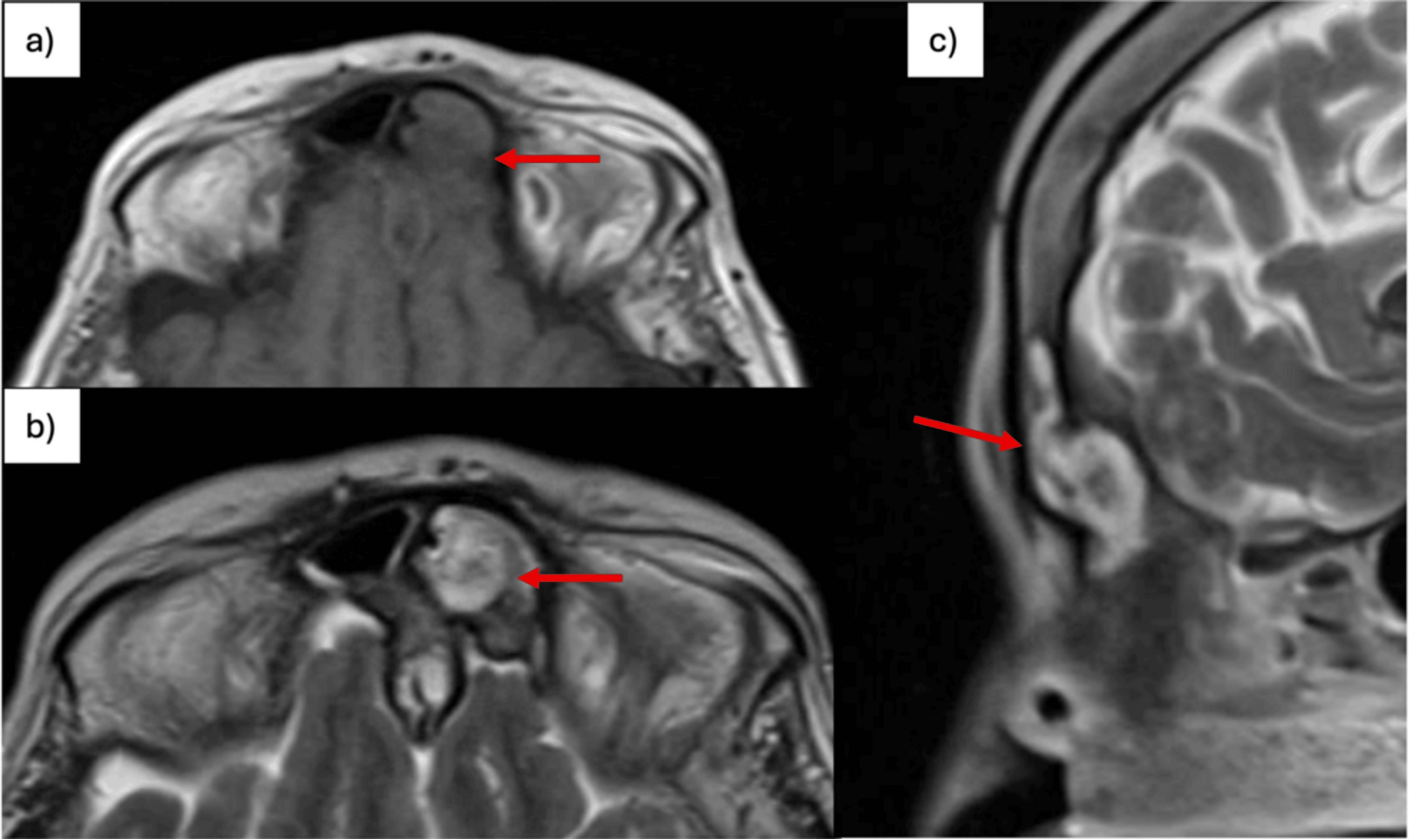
Cranial MRI (a) Axial T1-weighted image; (b) Axial T2-weighted image; (c) Sagittal T2-weighted image. The lesion is located in the anterior and inferior region of the interhemispheric falx, extending to the cribriform plates and the left frontal sinus, and measures approximately 13.5 mm in thickness and 62 mm along the craniocaudal axis (red arrows).
